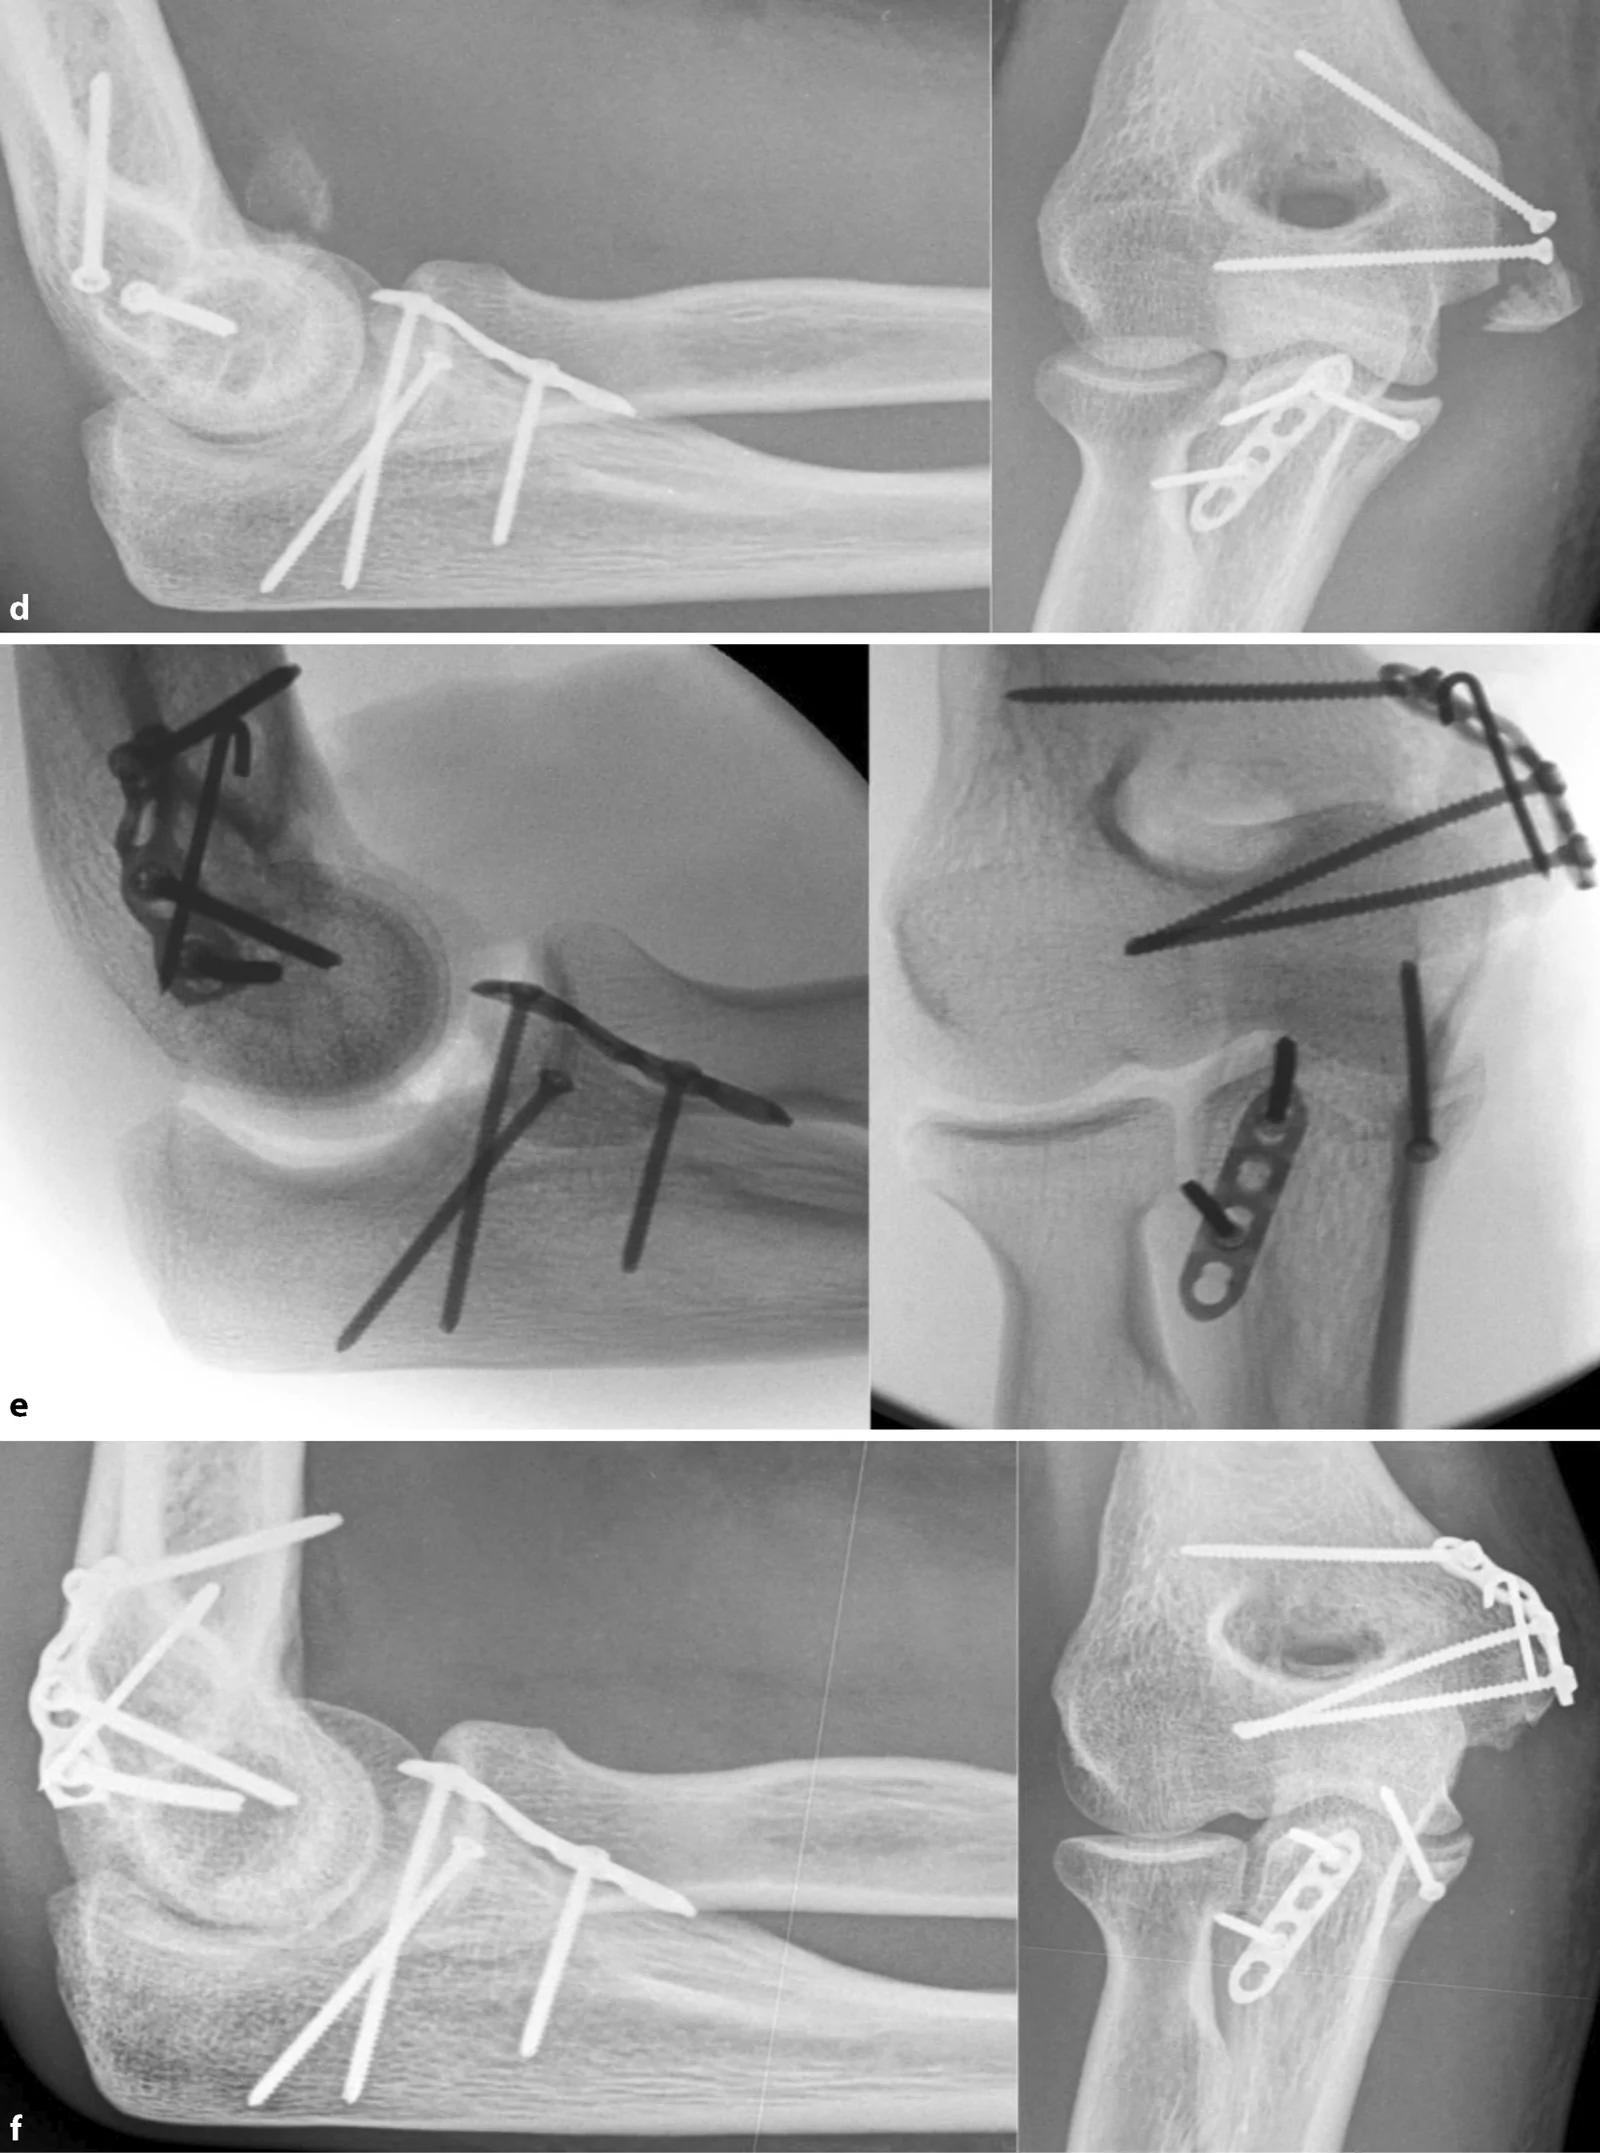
(Continued) Postoperative radiographs demonstrated loss of fixation of the medial epicondyle fragment (d). Revision fixation of the medial epicondyle was performed with a medial buttress plate. Intraoperative (e) and postoperative radiographs at 3 months (f) demonstrated stable fixation, with elbow range of motion from 155° of flexion to 0° extension and from 90° of pronation to 90° of supination
